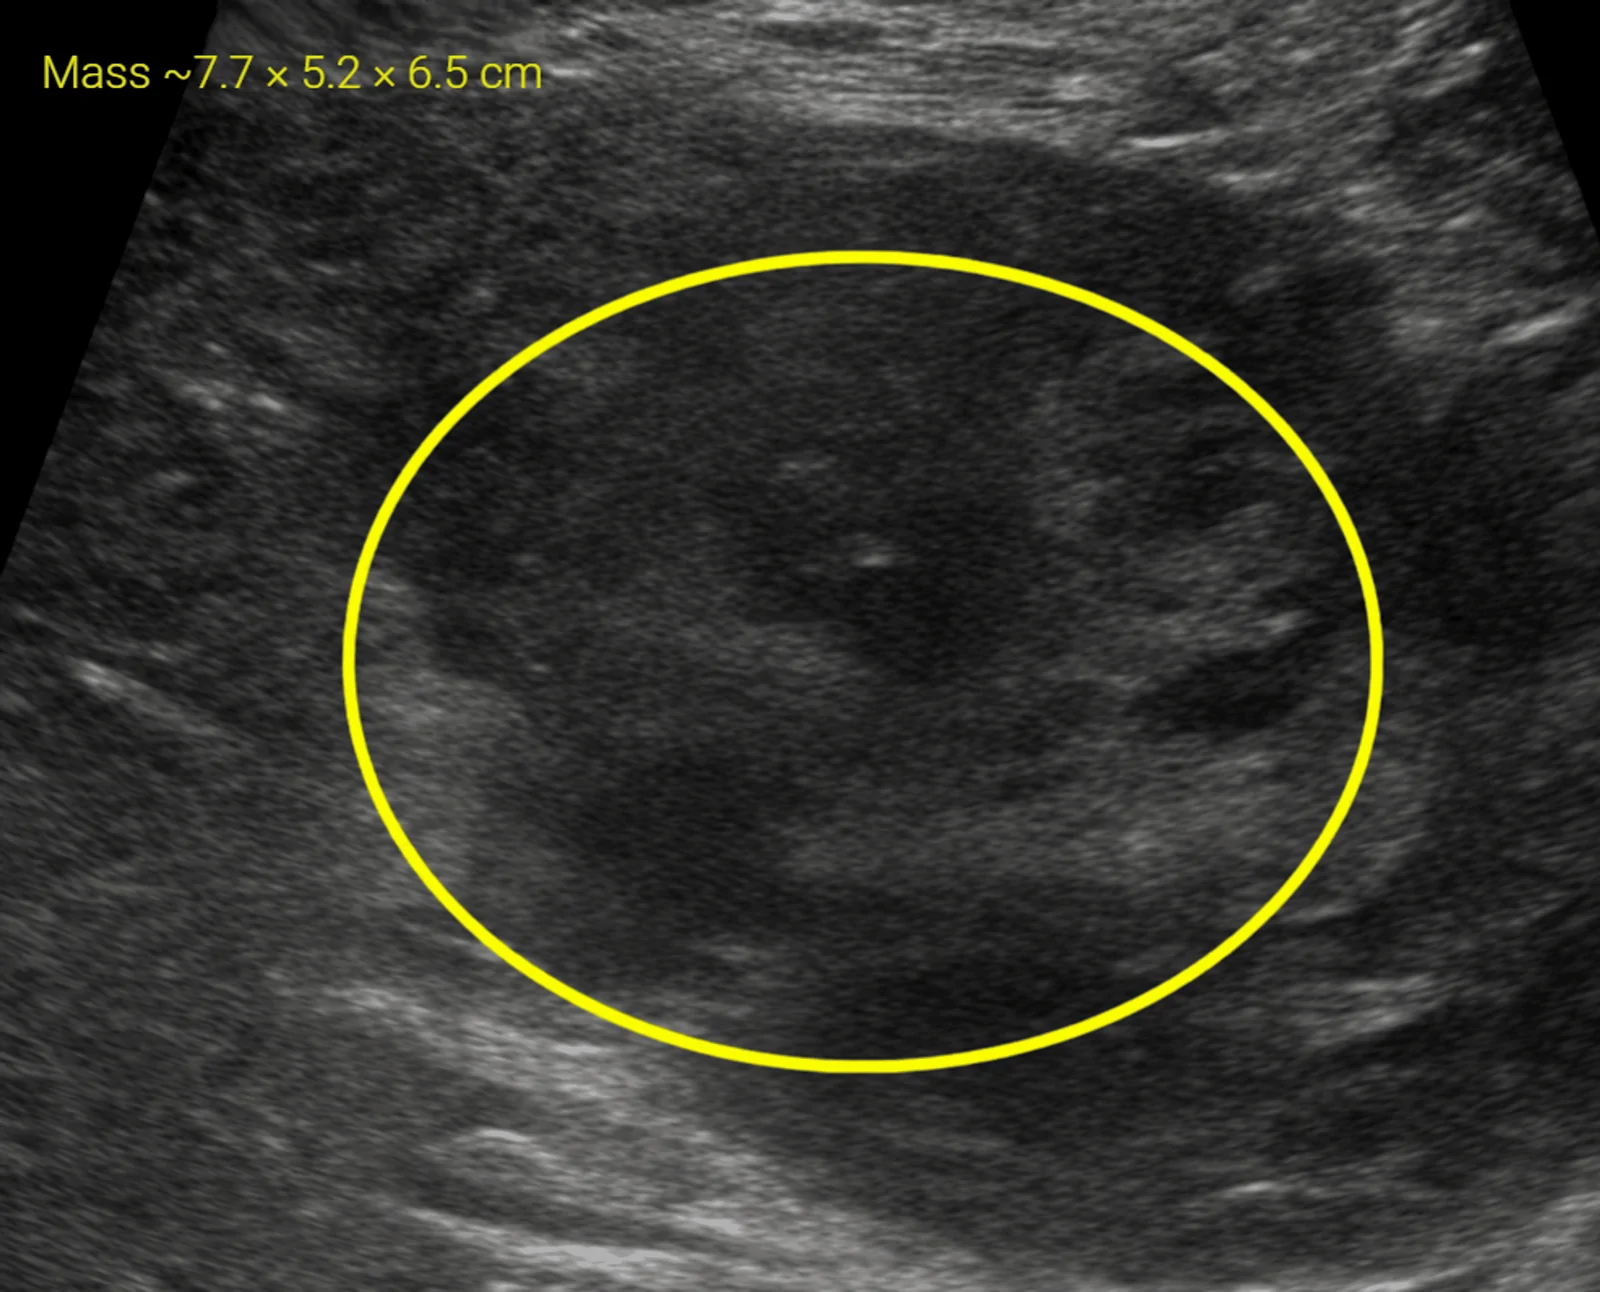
Grayscale ultrasound of the left thigh showing a large, heterogeneous, predominantly hypoechoic lesion (yellow circle).
